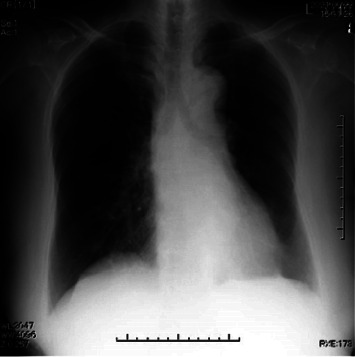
Chest radiograph on postoperative day 4.
